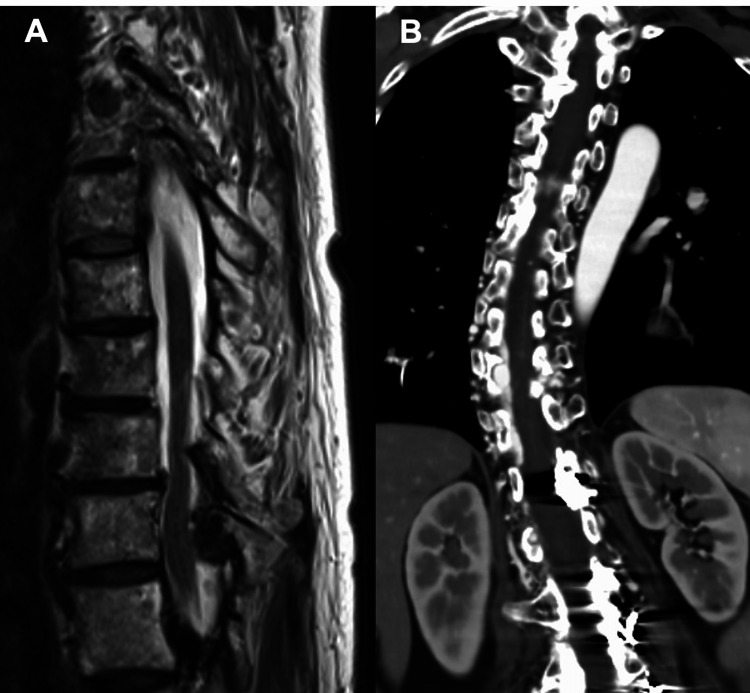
Thoracolumbar spinal MRI and coronary CTA findings after treatment A) Sagittal T2-weighted MRI showing improvement of spinal cord edema and flow voids. B) On arterial phase CTA, the previously observed spinal veins disappeared.
